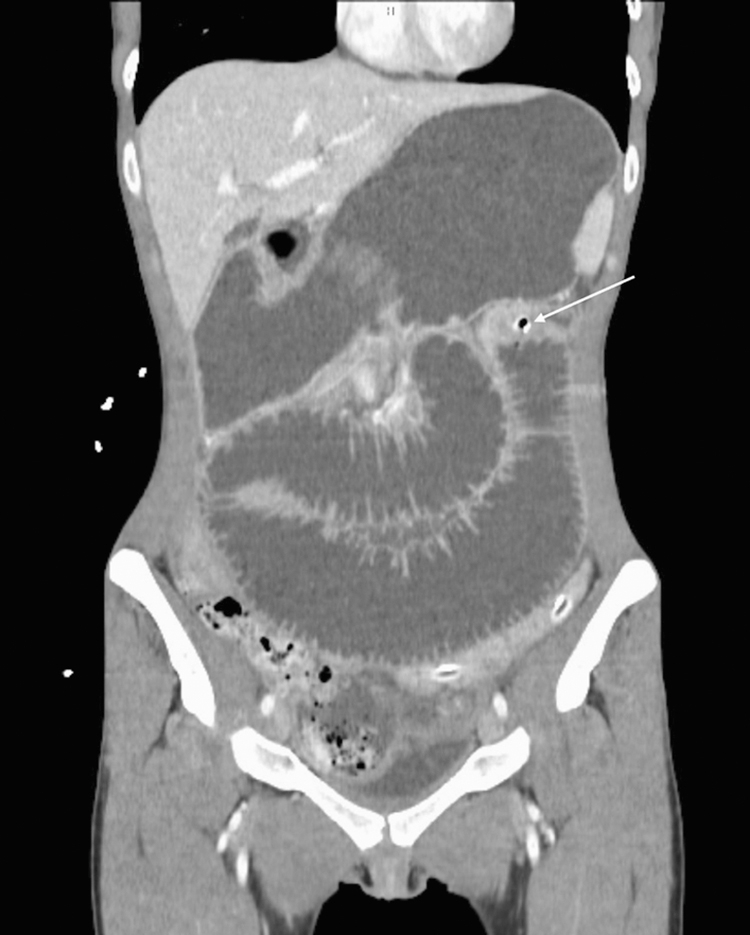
Coronal computed tomography demonstrating gastric and proximal small bowel dilation with a transition point associated with a jejunostomy tube balloon (arrow); jejunal loops measure up to 6 cm.
